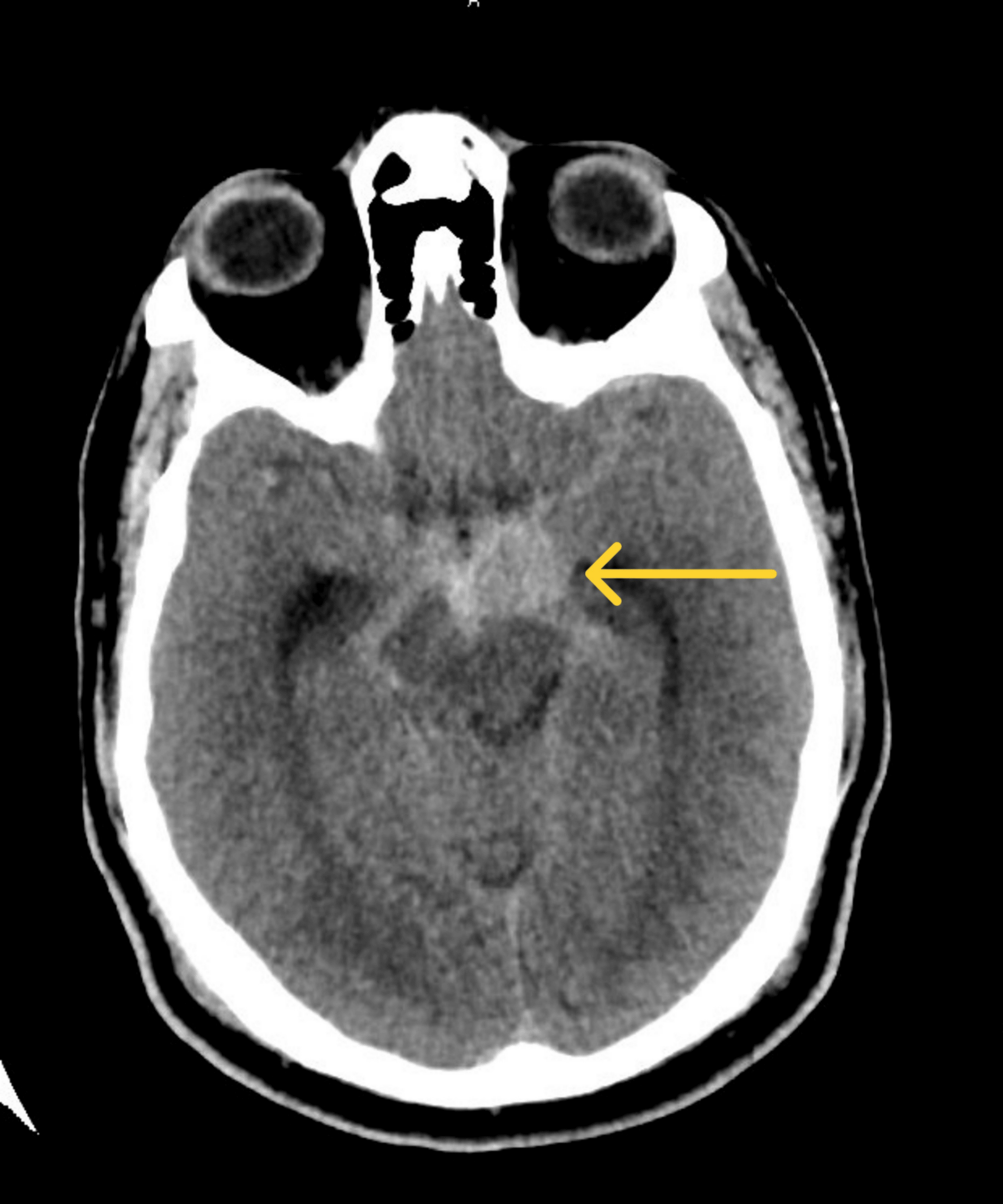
Head CT scan, axial view showing small saccular aneurysm in the M1 segment of the left middle cerebral artery and also an acute-subacute subarachnoid hemorrhage, with modified Fischer grade 1
